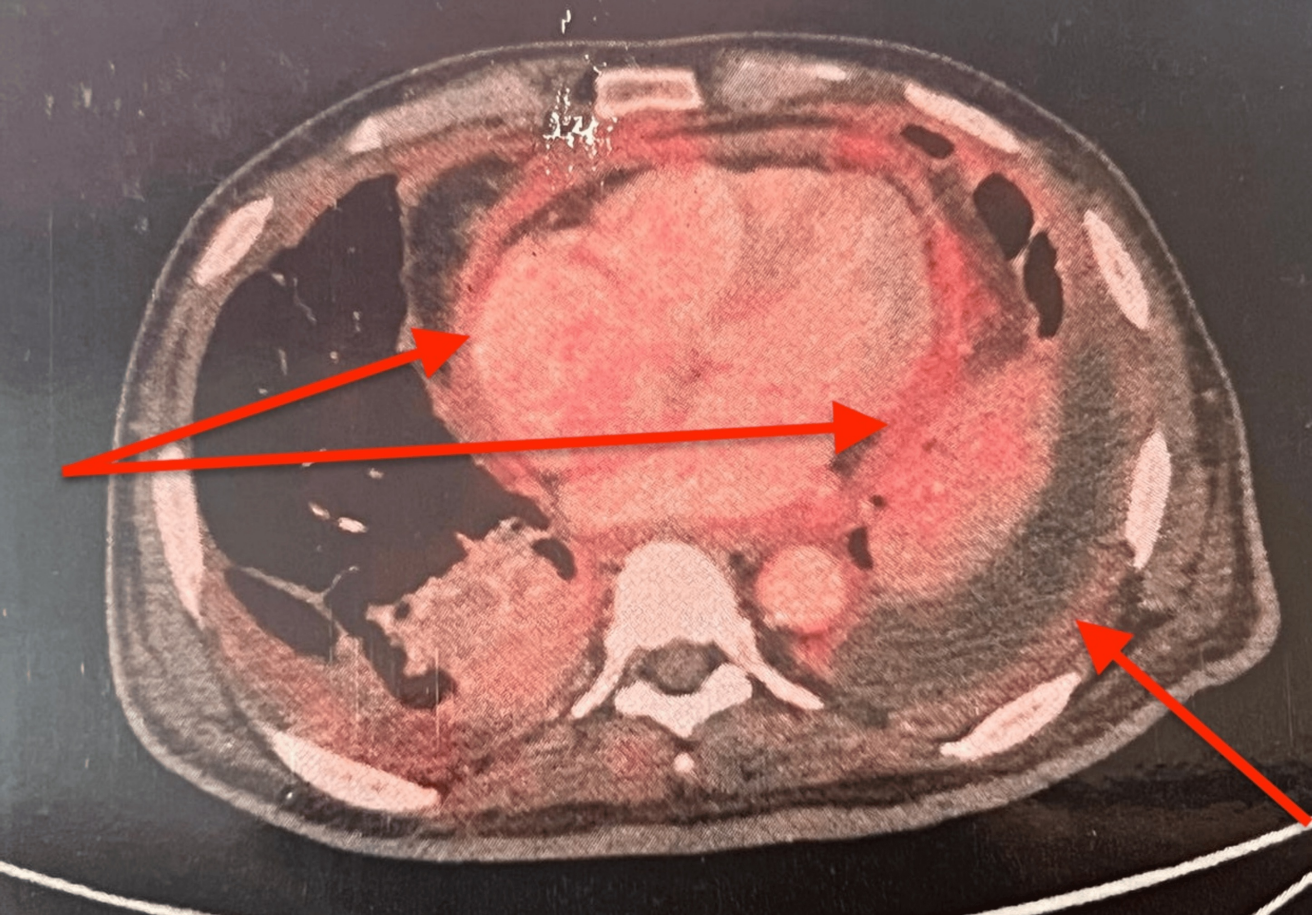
Low-grade FDG uptake with diffuse bilateral pleural thickening and effusion, pericardial thickening with effusion, and residual consolidation-collapse FDG: fluorodeoxyglucose
